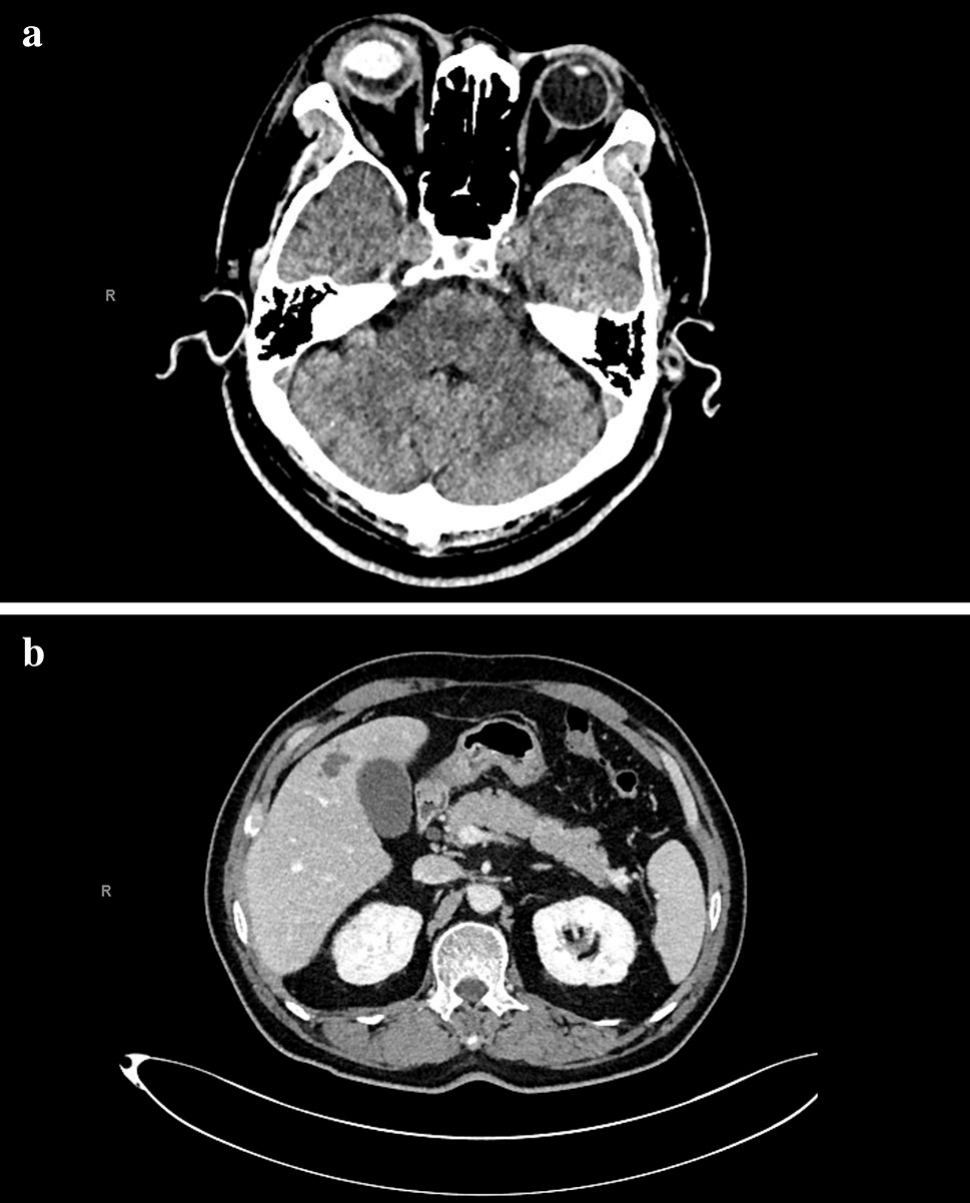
a CT scan of the Brain: diffuse thickening and blurred demarcation of right ocular walls. Exophthalmos associated with suffusion of glandular and periorbital adipose tissue. b CT scan of the Abdomen and Pelvis: hepatic non significantly vascularized lesion (25 × 14 mm) between 5 (V) and 8 (VIII) segments
